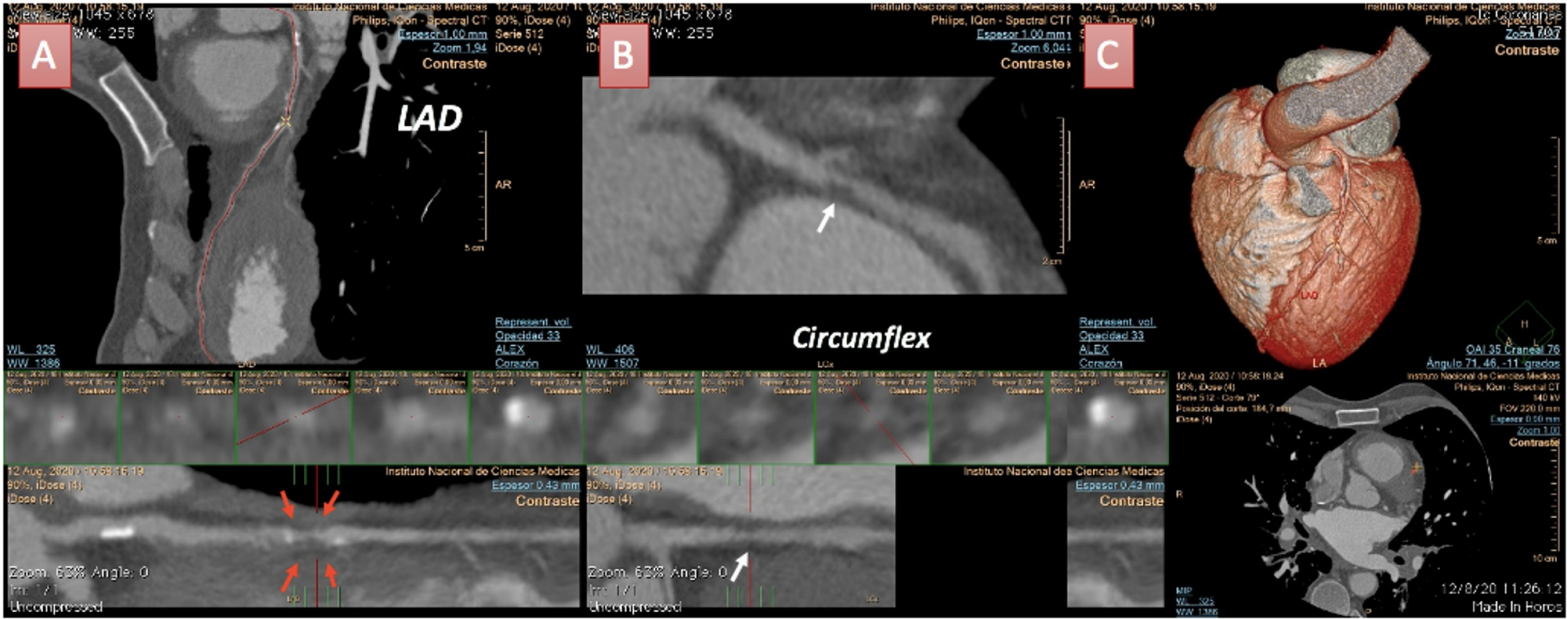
Coronary computed tomographic angiography findings of the patient. A: Left anterior descending (LAD) coronary artery in a curve multiplanar reconstruction (MPR) view with diffuse involvement and calcified plaques. Also, there was a large non-calcified plaque (red arrows) at the medial segment causing a significative obstruction (75-99%). B: Circumflex coronary artery in a curve MPR view showing a non-calcified plaque (white arrow) with non-significant obstruction (25-49%). C: 3D volume rendering of LV and coronary arteries distribution of an anteroposterior view.
